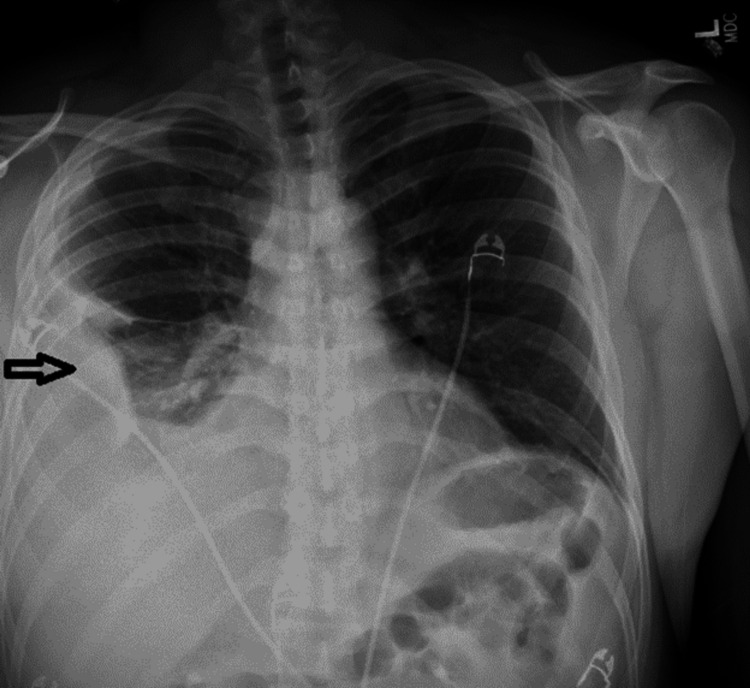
Admission chest X-ray showing a right-sided pleural effusion (arrow)
